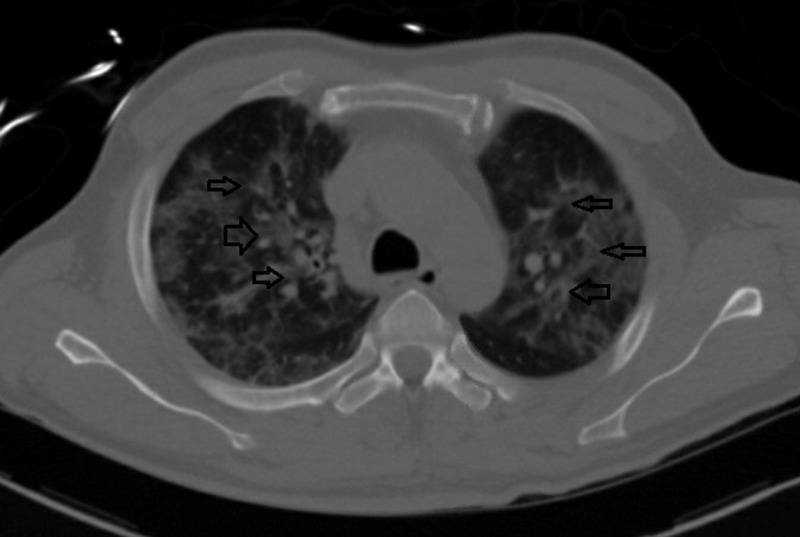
Chest CT shows diffuse bilateral ground-glass opacities/pneumonia compatible with COVID-19 infection (black arrows).
